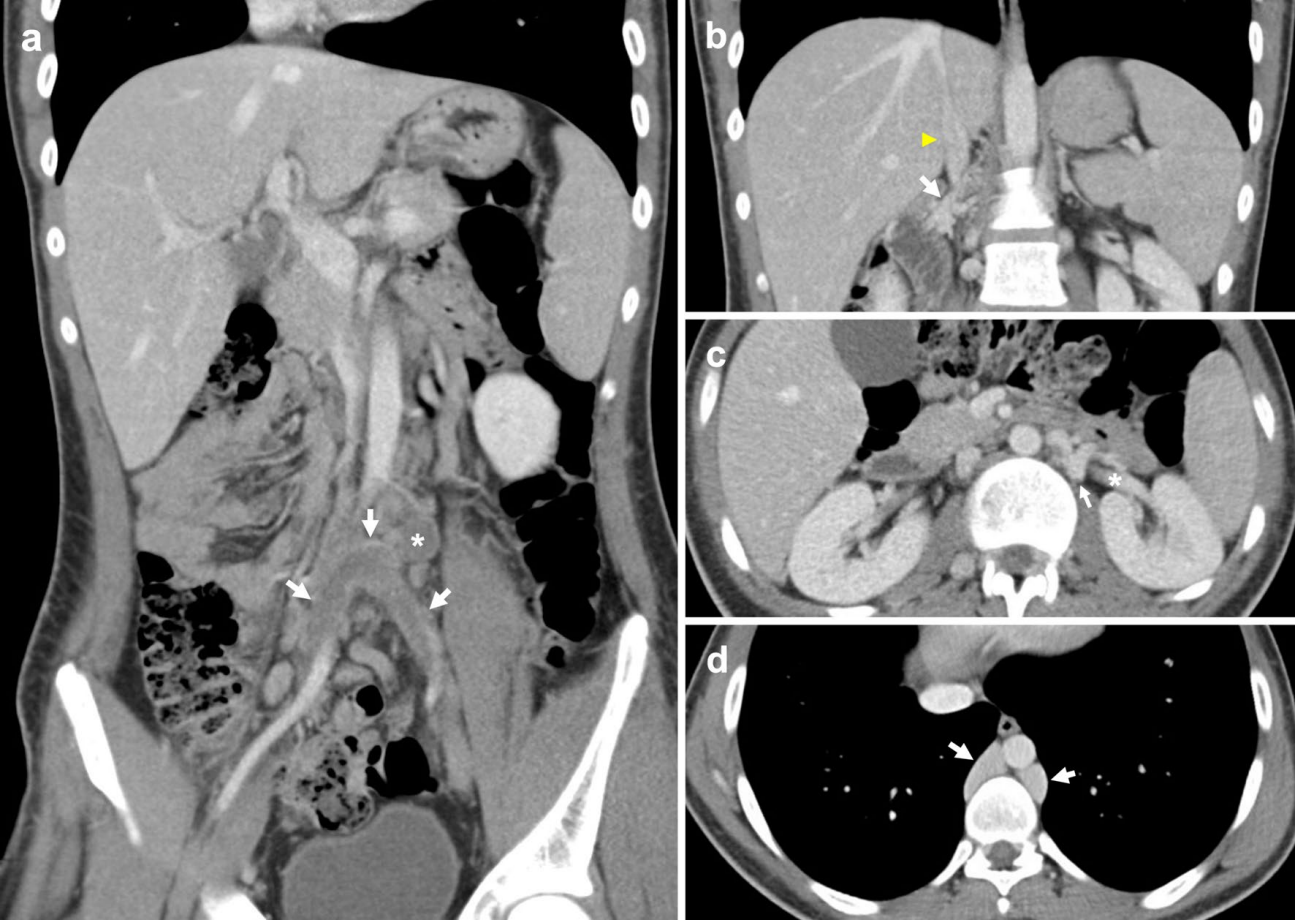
Extensive venous thrombus secondary to interrupted IVC with azygos continuation. A 19-year-old man presented with a right lower limb DVT and underwent contrast-enhanced CT to assess the proximal extent of thrombus. He later developed a right ankle ulcer, likely contributed to by post-thrombotic syndrome. a Coronal CT image in the portal venous phase shows occlusive venous thrombus (arrows) in the common iliac veins and an adjacent paravertebral collateral vein (asterisk). Infrarenal and renal segments of the IVC are absent. b Coronal CT demonstrates the right renal vein (arrow) draining into a small caliber interrupted inferior vena cava (arrowhead). c Axial CT shows a left renal vein (asterisk) that drains into a tortuous left-sided paravertebral collateral vein (arrow). d Axial CT image with distended azygos and hemiazygos veins (arrows)
